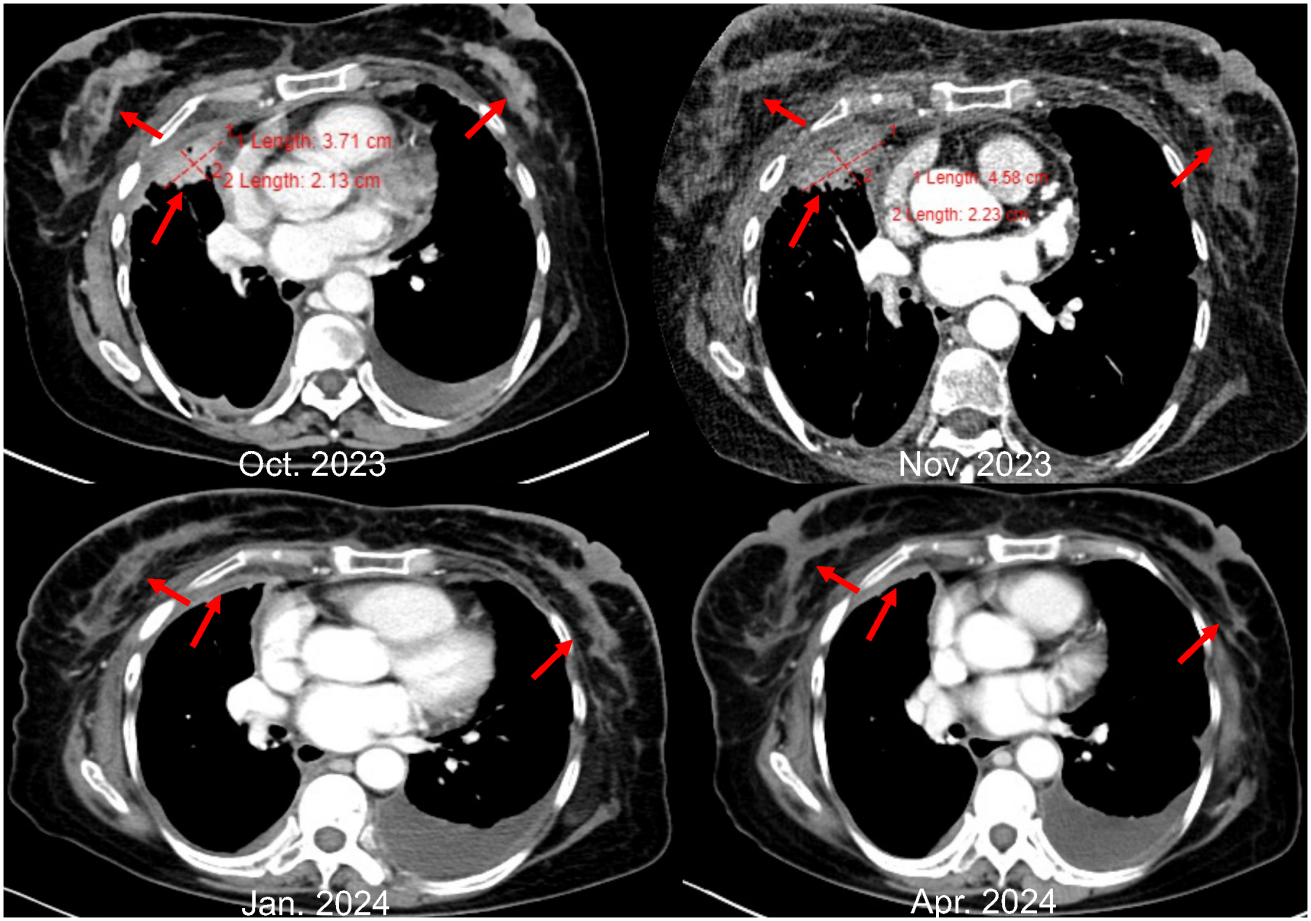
CT image changes of chest lesions.
